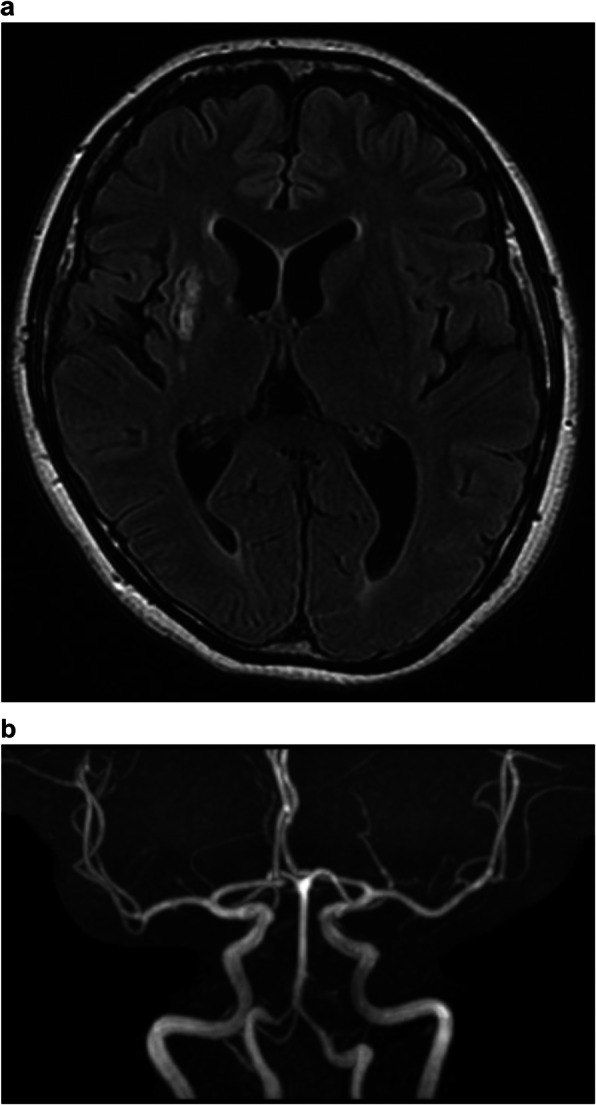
Brain magnetic resonance image at 11 months postoperatively (a-b) No cerebral infarction can be observed, and the major cerebral arteries are well visualized
